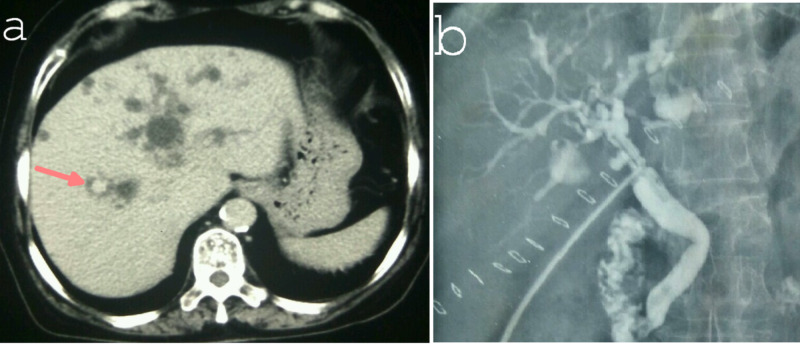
Contrast CT (a) and T-tube cholangiogram (b) showing type V choledochal cyst involving both the lobes of the liver. Note the characteristic “central dot sign” in the CT image of Caroli’s disease.
